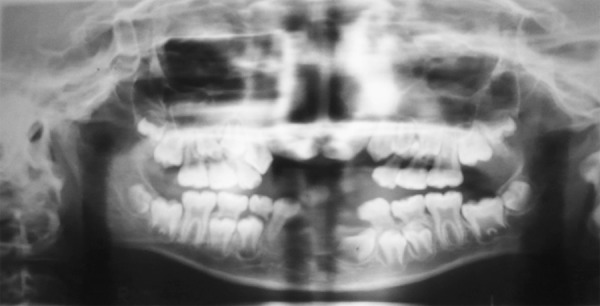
Radiograph showing absence of incisors
